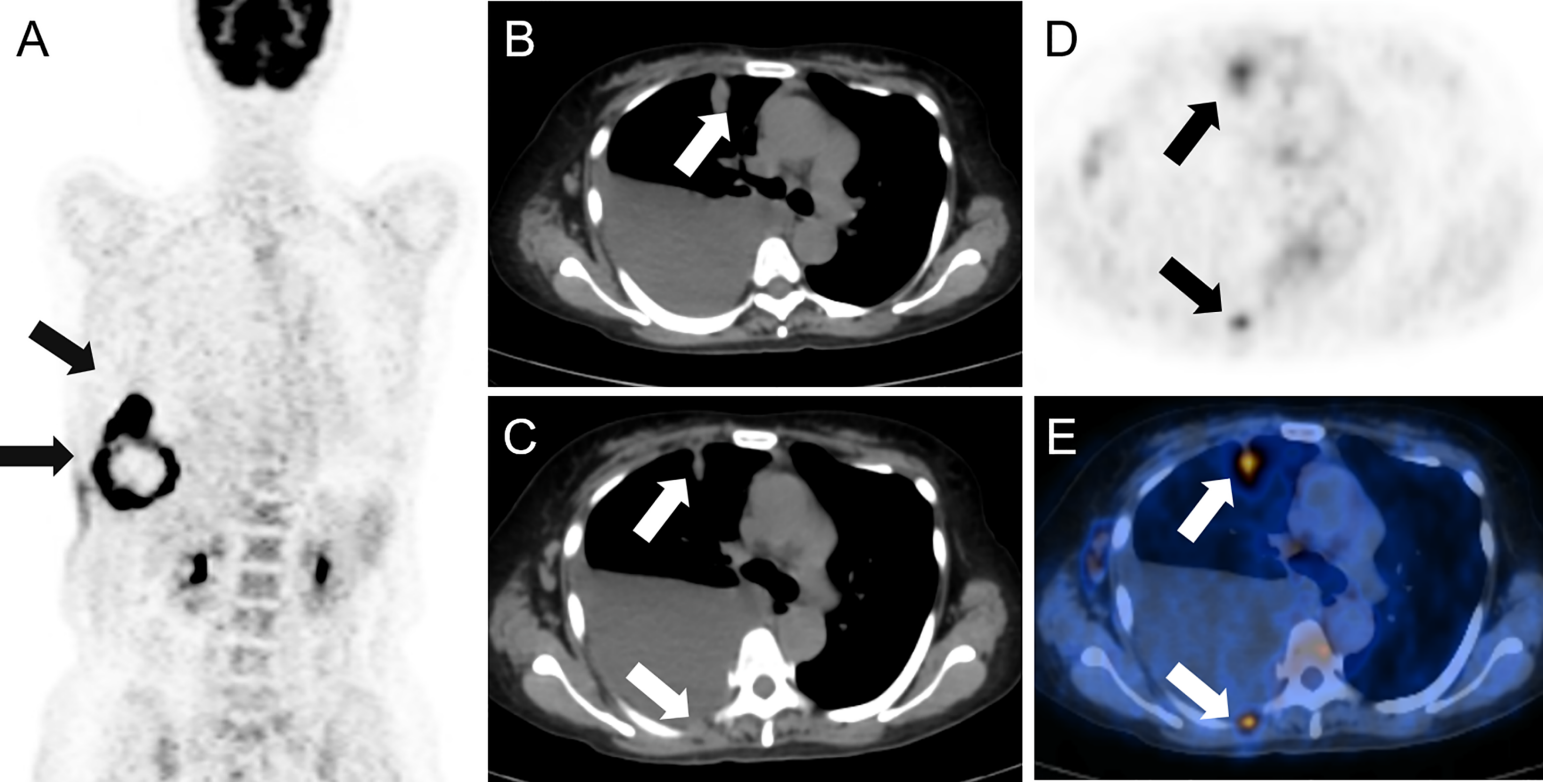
A large mass showing hypermetabolism peripherally and hypometabolism centrally was found on 18F-fluorodeoxyglucose positron emission tomography/computed tomography (A), arrows. Hypermetabolic lesions were imaged in the right lung and the posterior pleural wall (arrows) on axial computed tomography (B, C), positron emission tomography (D) and fusion imaging (E).
